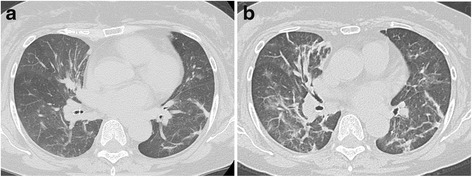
Chest computed tomography images. a Chest computed tomography on admission. b Chest computed tomography at the time of worsening of respiratory failure
